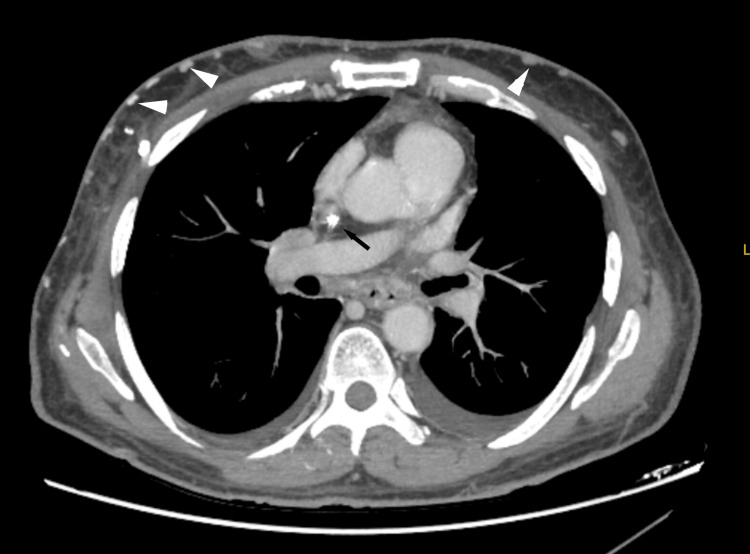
CT venography demonstrating extensive subcutaneous collateral vessels. Contrast-enhanced CT venography (axial view) demonstrates extensive subcutaneous collateral vessels (white arrowheads) in the anterior chest wall, consistent with SVC occlusion syndrome. Note the absence of contrast opacification in the expected location of the distal SVC (arrow), confirming complete occlusion at the catheter tip. SVC, superior vena cava; CT, computed tomography
